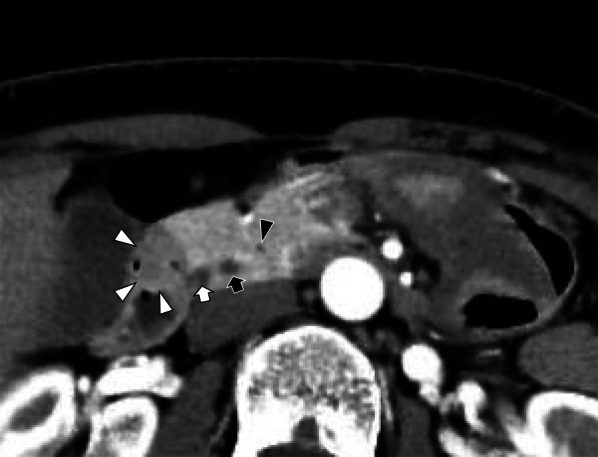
Abdominal contrast-enhanced CT scan. A well-defined 10-mm tumor was observed in the descending part of the duodenum at the border with the pancreas (white triangles). There was no dilatation of the main pancreatic duct (black arrow) and common bile duct (white arrow). The accessory pancreatic duct was identified (black triangle)
